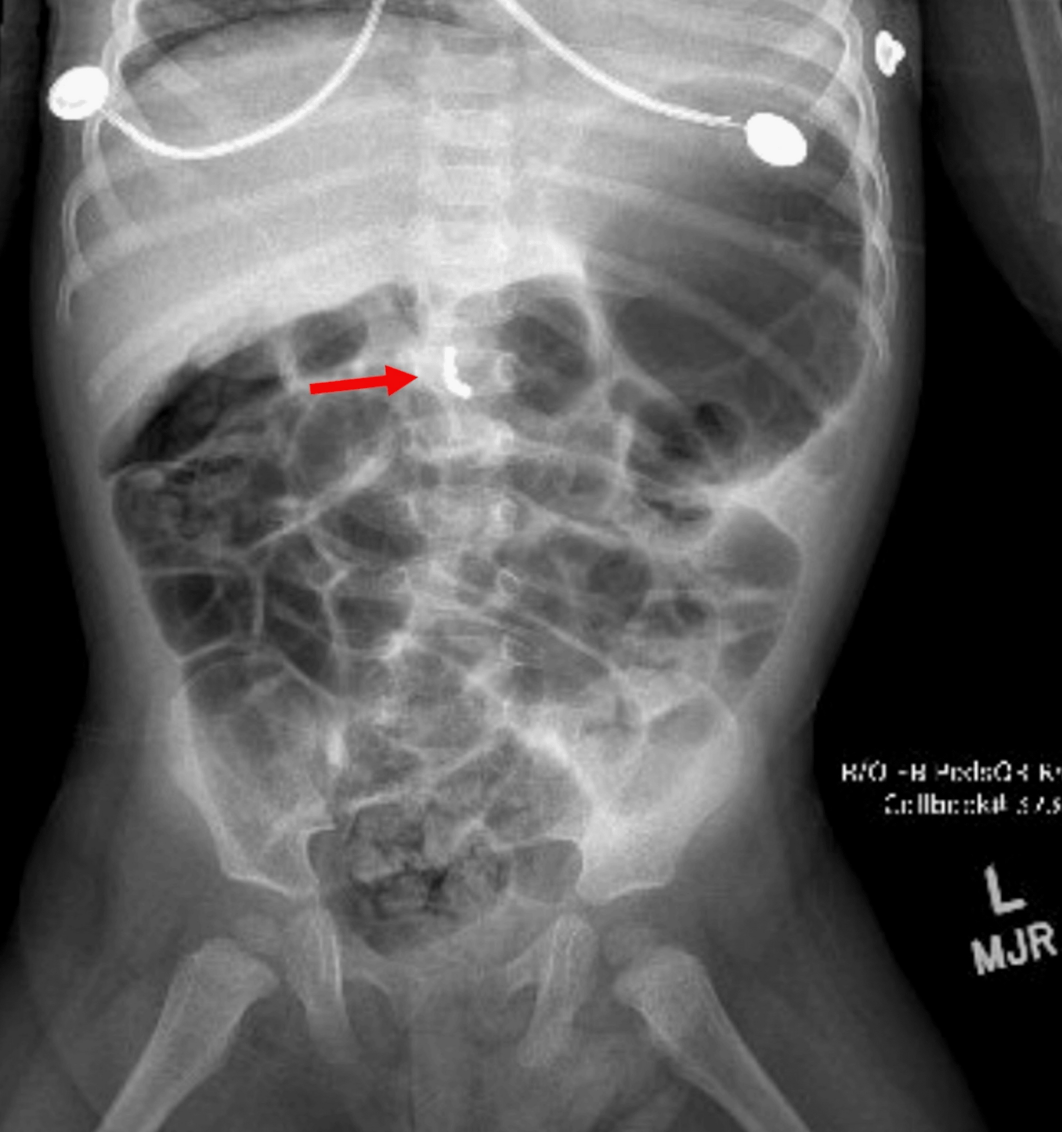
Intraoperative X-ray demonstrating magnetic beads (red marker) in the duodenum
